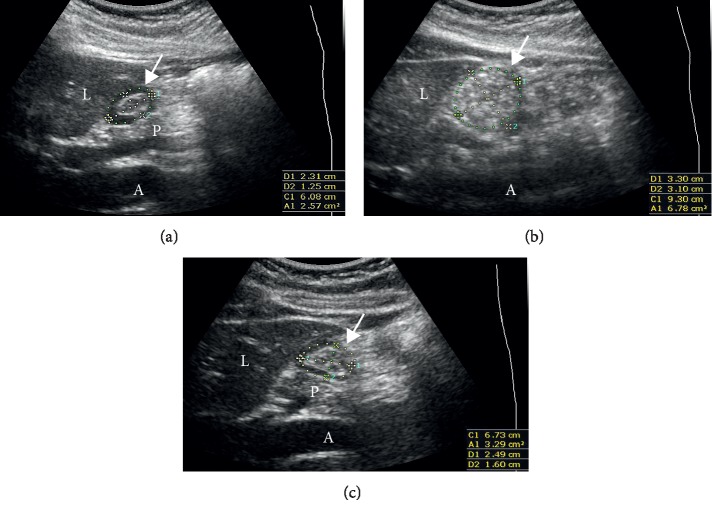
Representative ultrasonographic images of cross-sectional area of the gastric antrum (arrow) in the parasagittal plane: (a) at the time of baseline measurement, indicating fasting stomach, (b) 2 h after the intake of the rice-based meal, and (c) 6 h after the intake of the rice-based meal, indicating empty stomach. A = aorta; L = liver; P = pancreas.
